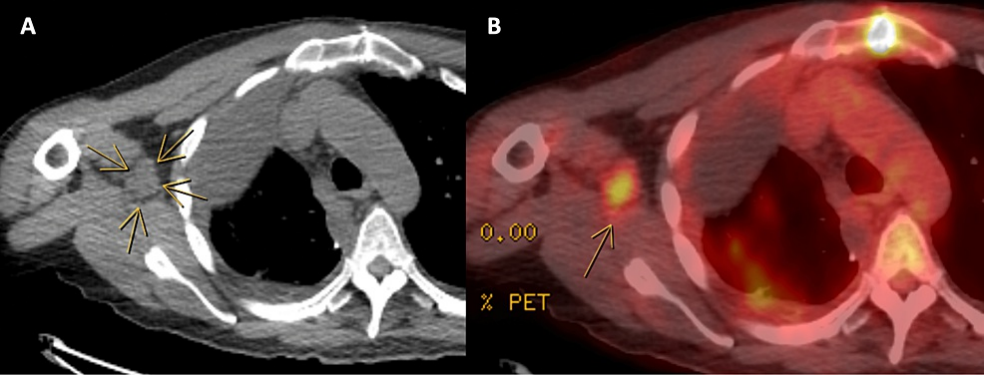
CT scan of tumor localization at five-month follow-up. Yellow arrows denote the residual axillary mass (A). PET scan of tumor localization at five-month follow-up. Yellow arrows denote the residual axillary mass (B). CT: Computed tomography; PET: Positron emission tomography.
